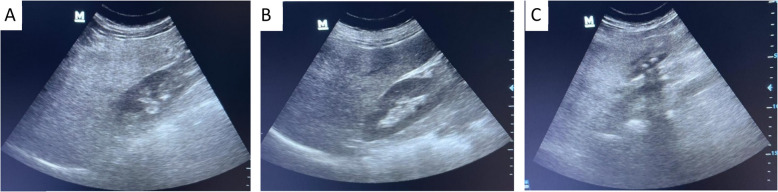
Ultrasound grading of hepatic steatosis based on liver echogenicity and visualization of key structures. A Grade 1: Slight and diffuse increase in liver echogenicity with normal visualization of the diaphragm and portal vein wall. B Grade 2: Moderate increase in echogenicity with slightly impaired visualization of the portal vein wall and diaphragm. C Grade 3: Marked increase in echogenicity with poor or no visualization of the portal vein wall, diaphragm, and posterior part of the right liver lobe. All three steatosis grades are represented in the figure
